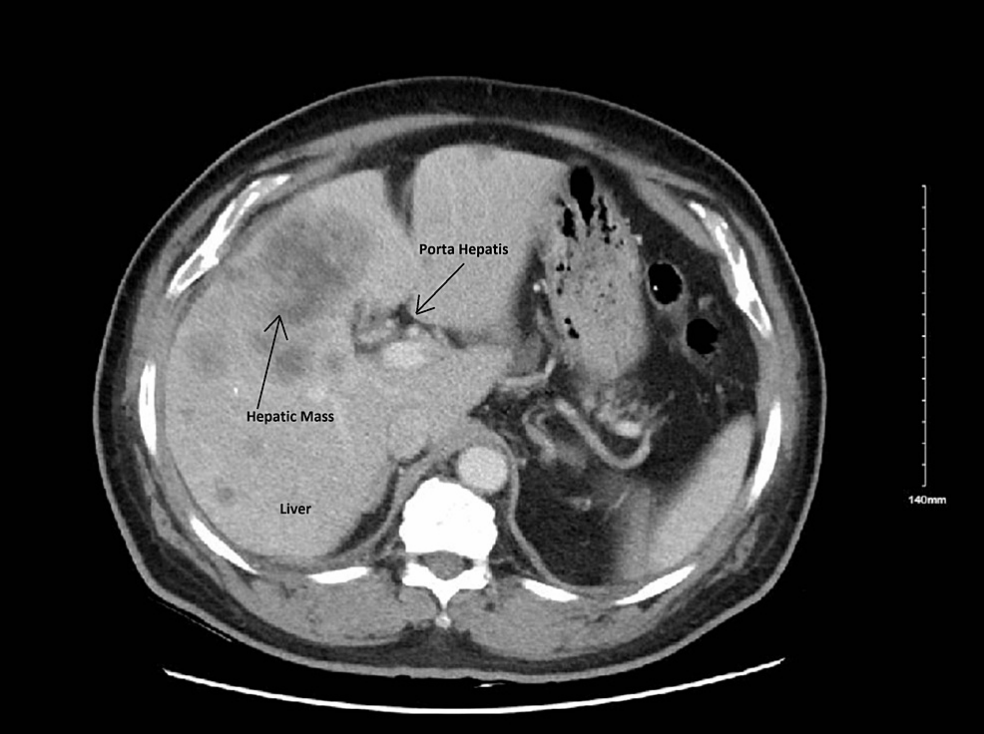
CT abdomen revealing multiple hepatic masses.
